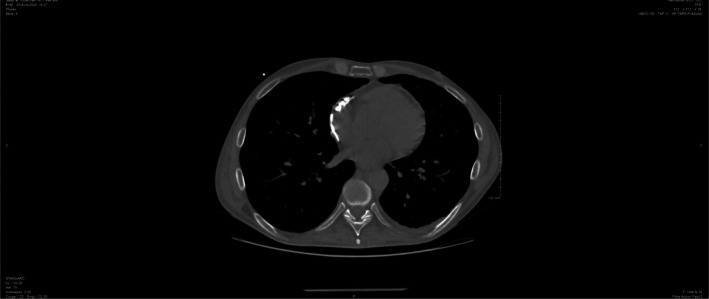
Echocardiographic section showing abundant pericardial effusion with compression of the heart CVAD.
